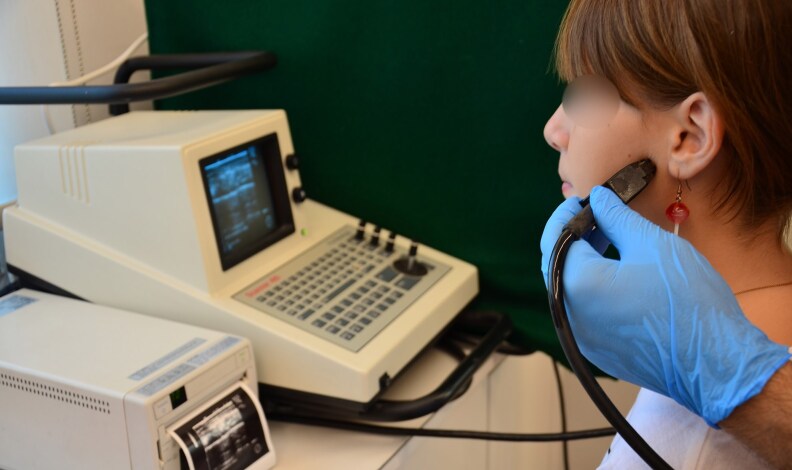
Ultrasound examination of the masseter muscle. A linear-array transducer (7.5 MHz) connected to a Pie Medical Scanner 480 was positioned over the masseter region, with the participant seated upright and the head maintained in a natural posture.
